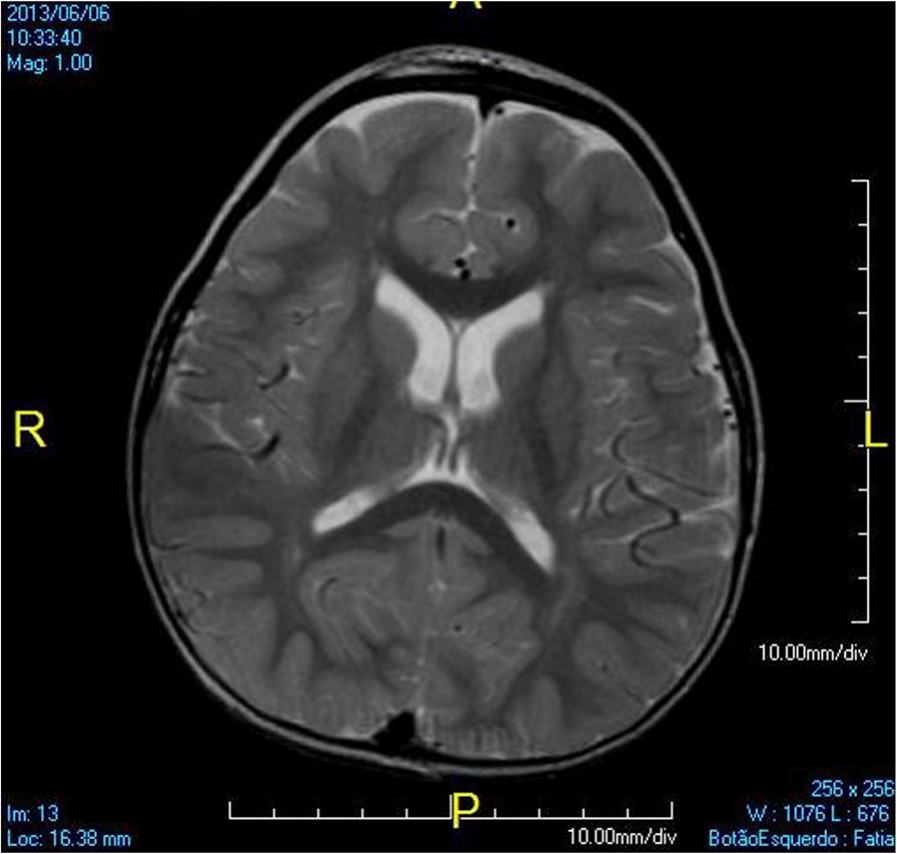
Brain MRI - Increased signal in T2-weighted images in the withe matter territories adjacent to the lateral ventricles bodies and subcortical zone
